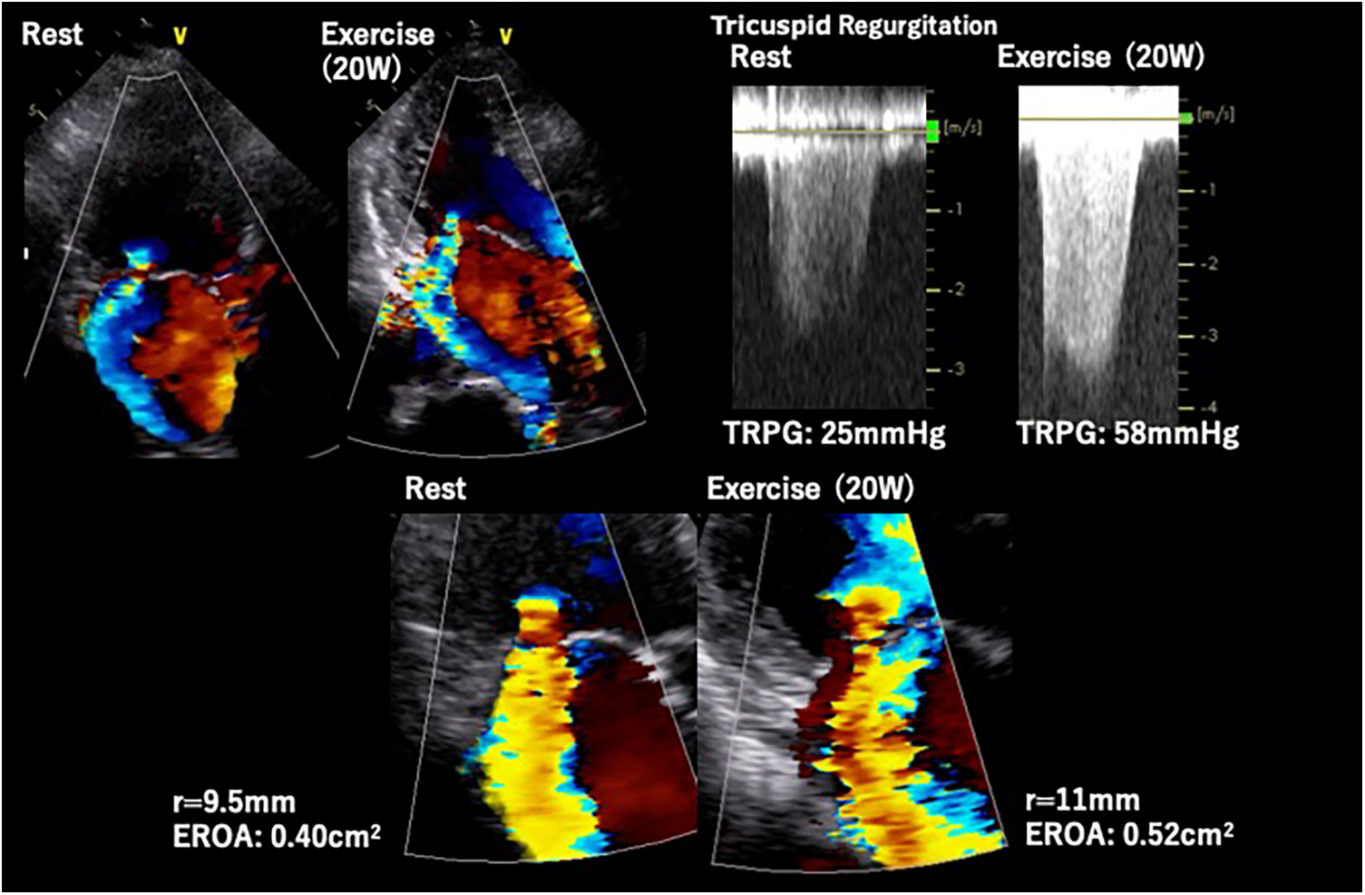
Exercise stress echocardiography for secondary mitral regurgitation. Seventy-eight-year-old man, a case of symptomatic secondary MR induced by non-ischemic cardiomyopathy. The MR severity changed from moderate to severe and quantitative parameters (EROA, RV, and SPAP) worsened during exercise. EROA, effective regurgitation orifice area; MR, mitral regurgitation; RV, regurgitation volume; SPAP, systolic pulmonary arterial pressure.
